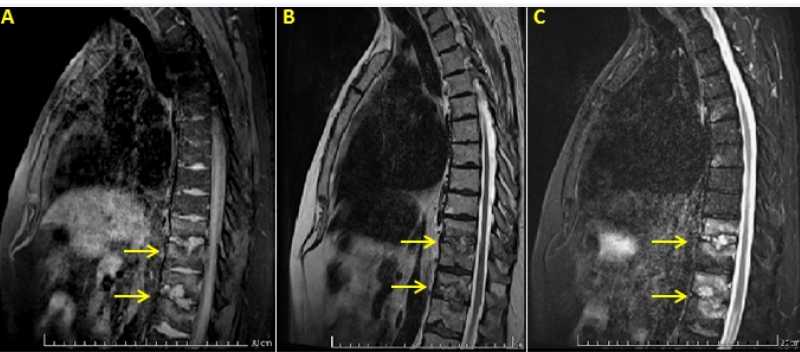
Magnetic resonance imaging with gadolinium infusion of the lumbar spine in T1 sequence (A), T2 sequence (B) and short TI inversion recovery (STIR) mode (C). The findings include diffuse disc edema and inflammation between T10 and T11 and T12 and L1 vertebral bodies (arrows), with no abscess or significant reduction in the height of the vertebral bodies.
